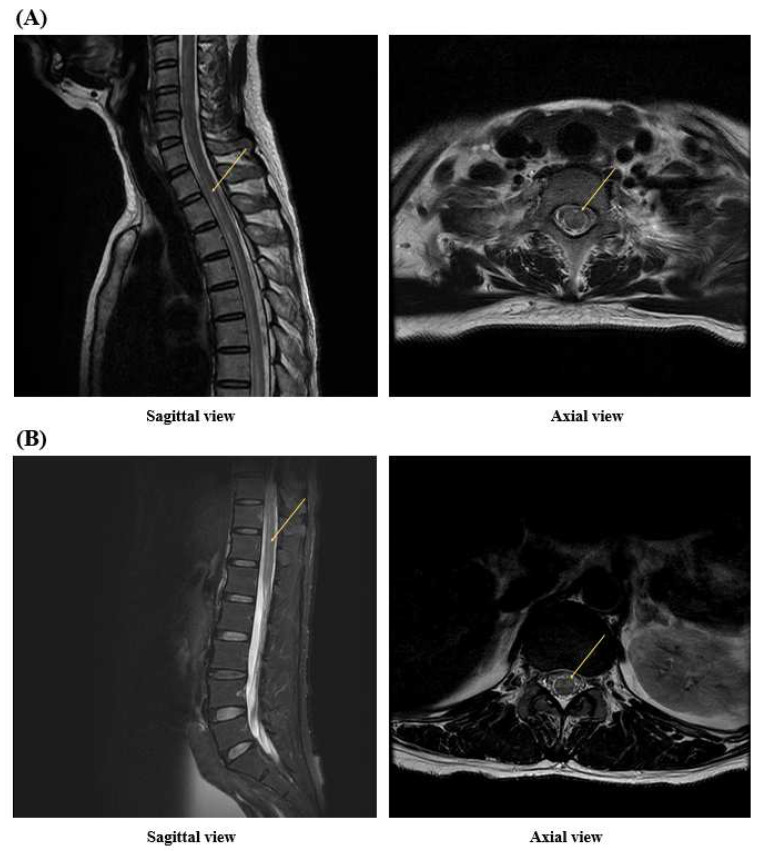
In (A), arrows indicates high signal intensity starting from the T2 vertebral body segment in the T2-weighted MRI image. In (B), arrows indicates high signal intensity in the T12 vertebral body segment on the T2-weighted MRI image. Longitudinally extensive transverse myelitis (LETM) involves three or more vertebral body segments. In the patient above, the lesion started from the T2 vertebral body segment and spanned 12 segments up to the T12 vertebral body segment. This is an important imaging finding in diagnosing NMOSD.
